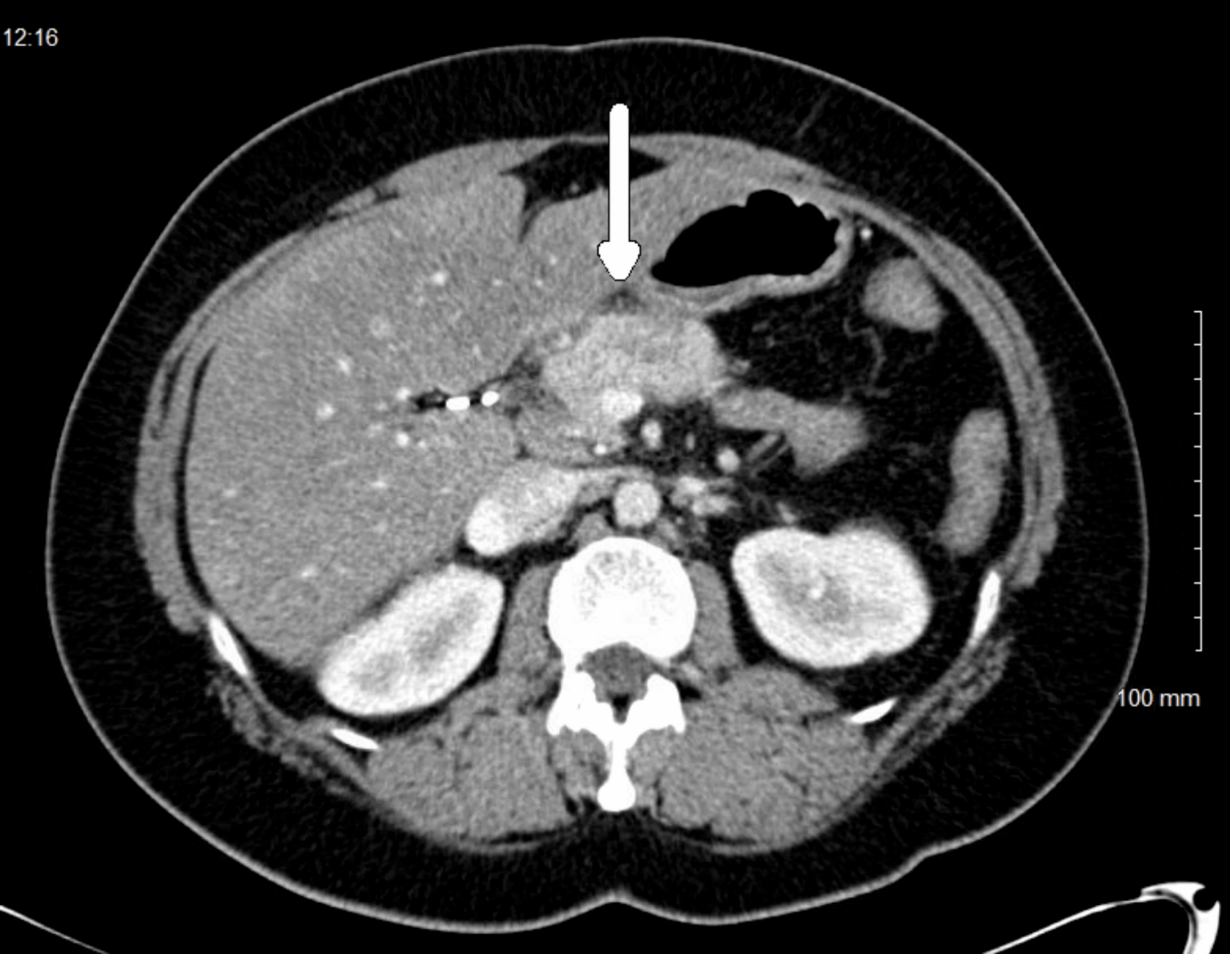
The CT showing a large enhancing mass in the pancreas (white arrow) CT: computed tomography
